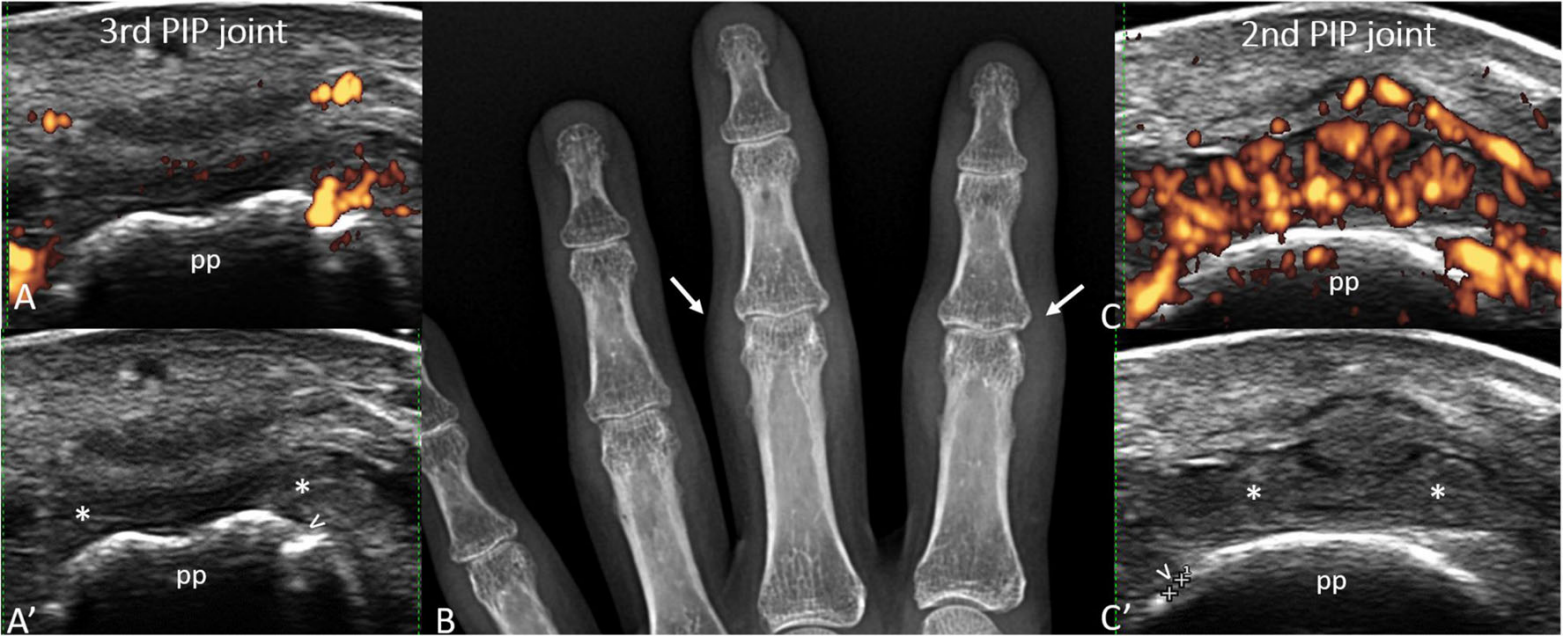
SLE-arthritis. Transverse ultrasound (US) scans of the dorsal aspect of 3^rd^ (A-A’) and 2^nd^ (C-C’) proximal interphalangeal joints, with (A and C) and without (A’ and C’) power Doppler mode, acquired using 10-22 MHz probe. US images show the presence of small bone erosions (arrowheads) and synovial hypertrophy (asterisks) with power Doppler signal (red spots). Soft tissue swelling (arrows), but no bone erosion, is visible on conventional radiography (B). Legend. Calipers: 0.48 mm bone erosion; pp = proximal phalanx
